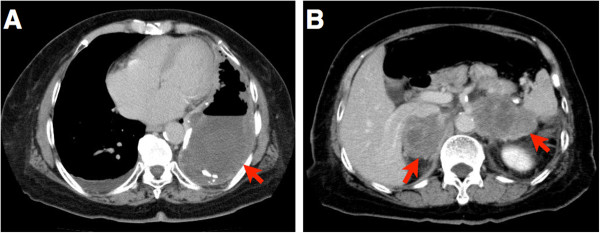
Enhanced computed tomography scan of the chest and abdomen. (A) Thoracic CT scan displayed large pyothorax and effusion in the left pleural cavity (indicated by a red arrow). (B) Abdominal CT scan displayed bilateral adrenal tumors (indicated by red arrows).
